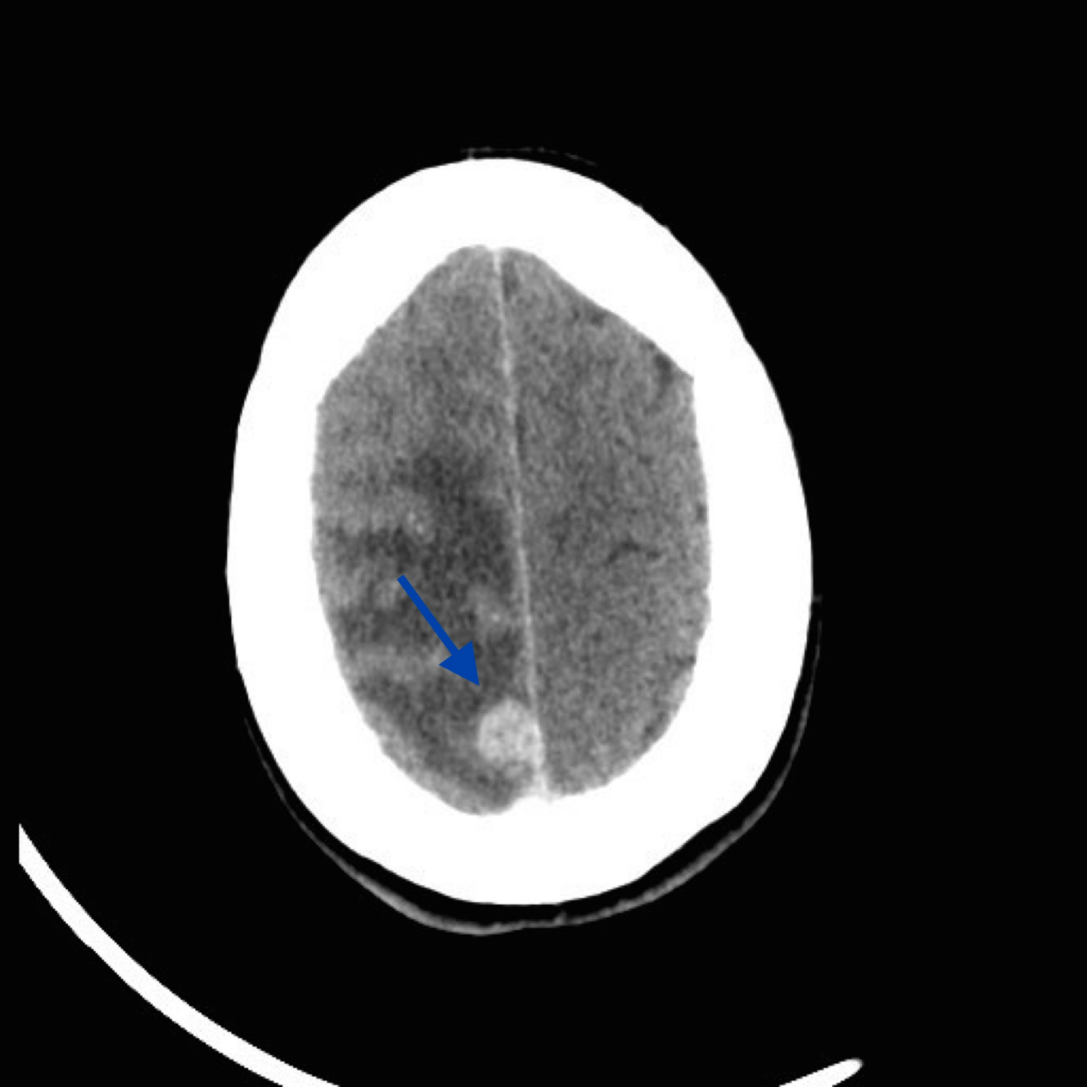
Preoperative axial CT scan with contrast: homogeneously enhancing parafalcine lesion in the right parietal region with surrounding vasogenic edema CT: computed tomography
